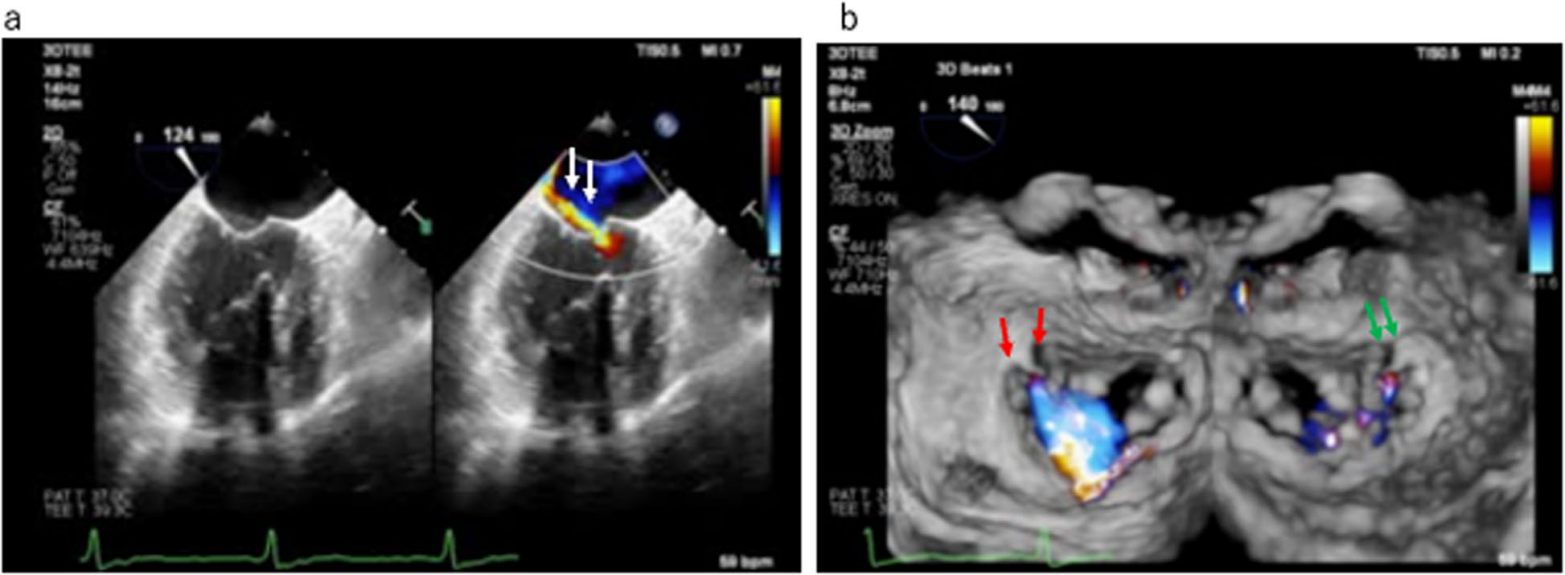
a Mid-esophageal long-axis view with a counterclockwise rotation of the probe and b 3-D color Doppler image in case 2. a Regurgitation jets apparently from an orifice in the center of the anterior leaflet (white arrows). b Regurgitant jets (red arrows) and the regurgitation orifice (green arrows) attributed to the prolapse of the anterior commissure (left: enface view, right: enface view from the left ventricular side)
